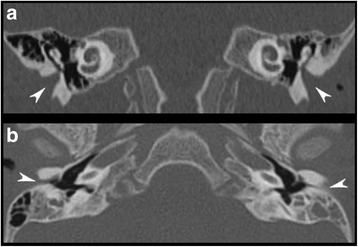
Temporal bone CT. Coronal (a) and axial (b) images reveal marked stenosis and peculiar vertical orientation of the bony portions of the external auditory canals (arrowheads) associated with rotation and fusion of the malleus to the lateral middle ear cavity wall
